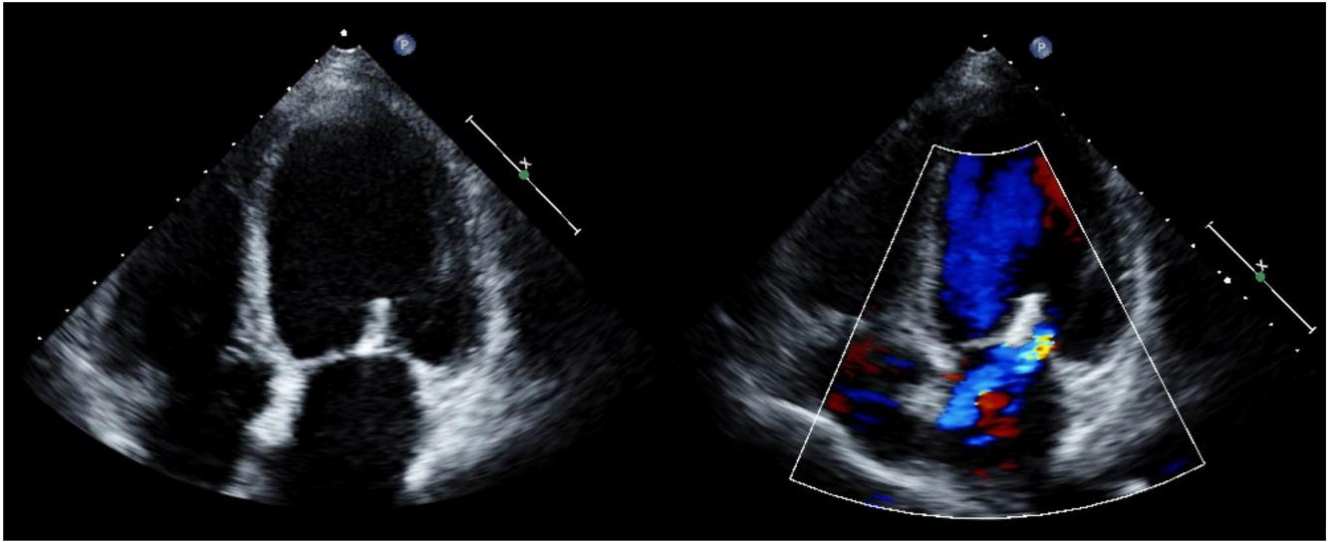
Transthoracic echocardiographic 6 months after TMVr findings. Left ventricular wall thickness and oedema have improved, and the MR is well-controlled.
